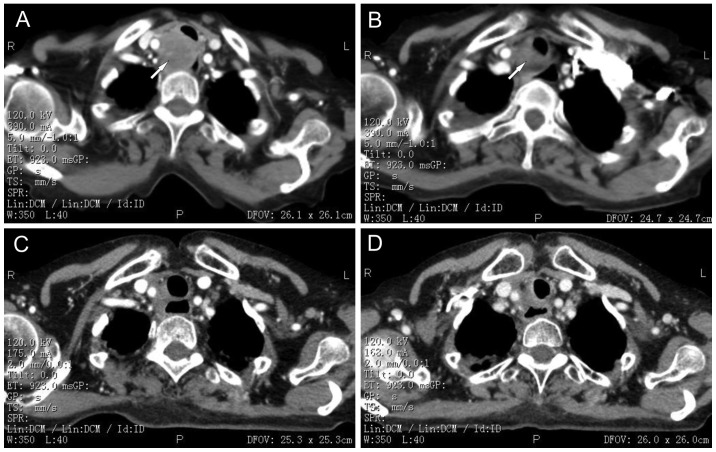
Enhanced computed tomography (CT) scans of the patient during treatment. (A) Enhanced CT scan demonstrating a paratracheal lymph node metastasis, 4.5×3.5×2.5 cm (white arrow), squeezing the trachea and esophagus. (B) Enhanced CT scan demonstrating the lesion reduced in size to 3.0×2.5×1.5 cm (white arrow) 1 month following a cycle of chemotherapy combined with endostar. Objective evaluation indicated partial remission. (C) Enhanced CT scan 1 month following stereotactic radiotherapy treatment. Objective evaluation indicated near complete remission. (D) Enhanced CT scan 4 months following radiotherapy and four cycles of chemotherapy combined with endostar. Objective evaluation indicated complete remission.
